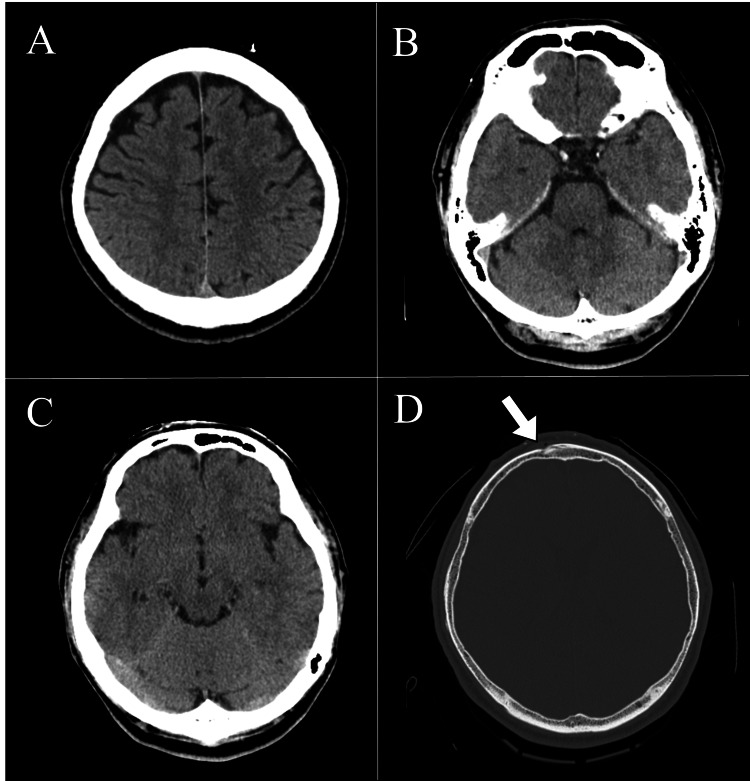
Head CT findings on the day of injury (day X). A–D represent axial images at different levels. No obvious hemorrhagic lesions were observed in the intracranial space on any of the sectional images. A: High parietal level. B: Pons level. C: Midbrain level. D: Fracture level, with the white arrow indicating the fracture site of the frontal bone.
